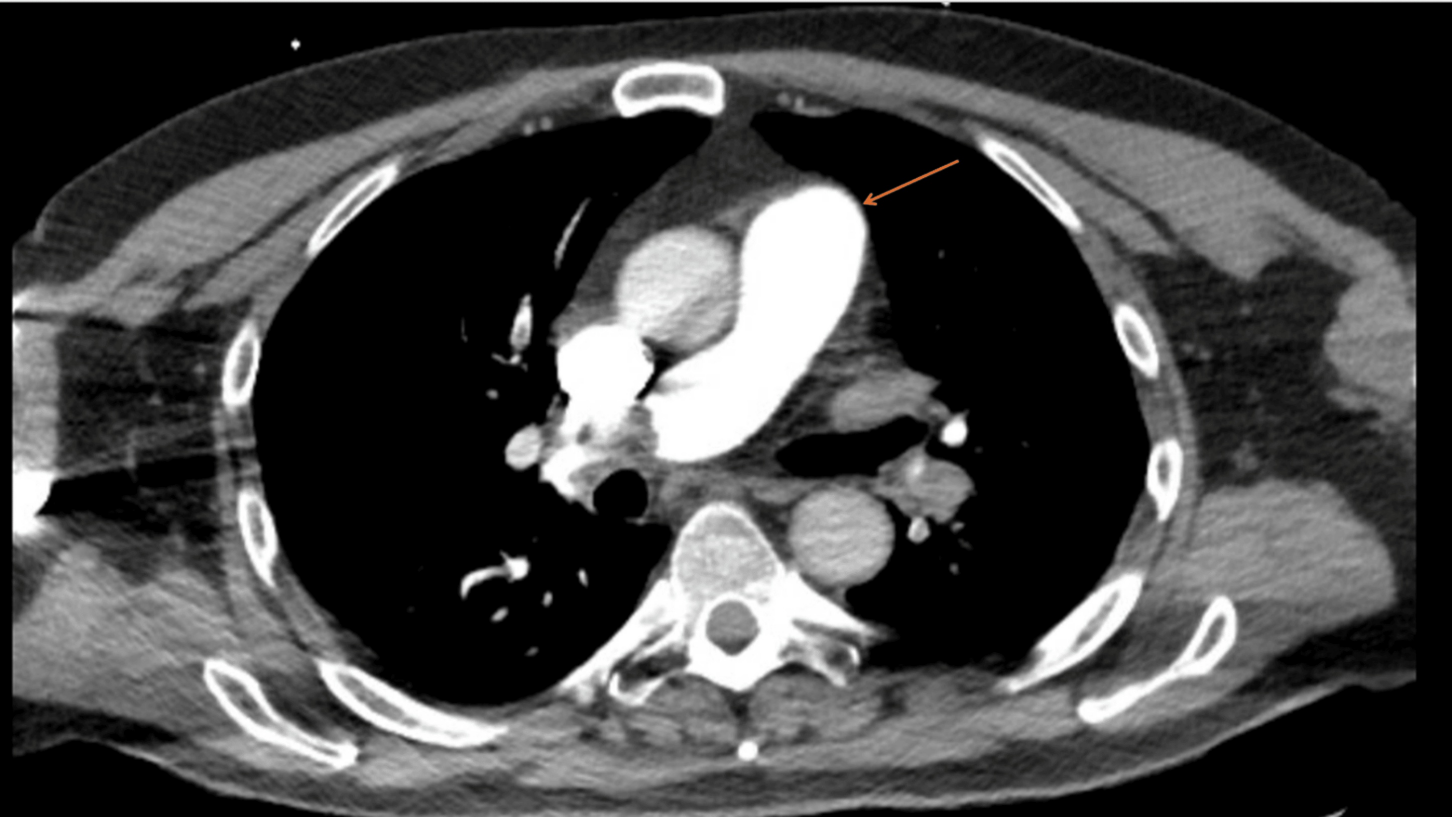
CT angiography of the chest demonstrating saddle pulmonary embolism (arrow) The arrow indicates a thrombus within the main pulmonary artery consistent with a saddle pulmonary embolism.
